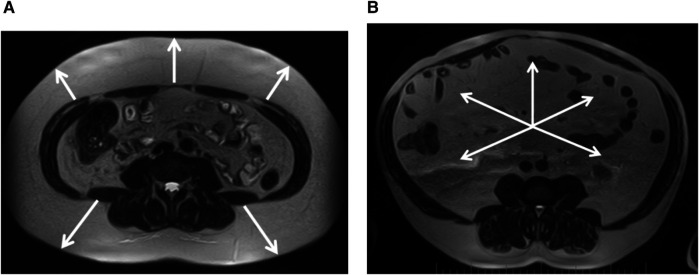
(A) Female, 39 years old, pronounced predominance of the subcutaneous adipose tissue (white arrows), VSR = 0.33. (B) Male, 57 years old, marked predominance of visceral adipose tissue (white arrows), VSR = 2.21. Magnetic resonance imaging of abdominal adipose tissue/abdominal fat. Axial T2-weighted turbo spin echo images at 4-5 lumbar vertebrae levels.
